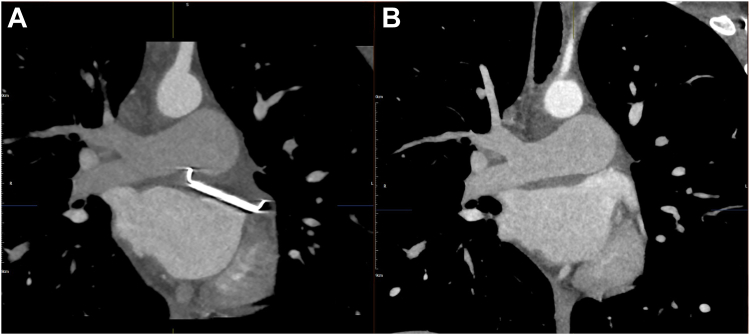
Oblique coronal computed tomography cardiac angiogram demonstrating left atrial appendage (LAA) closure. A*:* Postclosure: a radiopaque surgical clip is seen at the LAA ostium with complete absence of contrast opacification, confirming successful exclusion. B Preclosure: the LAA is fully opacified with contrast, demonstrating patency and communication with the left atrium.
